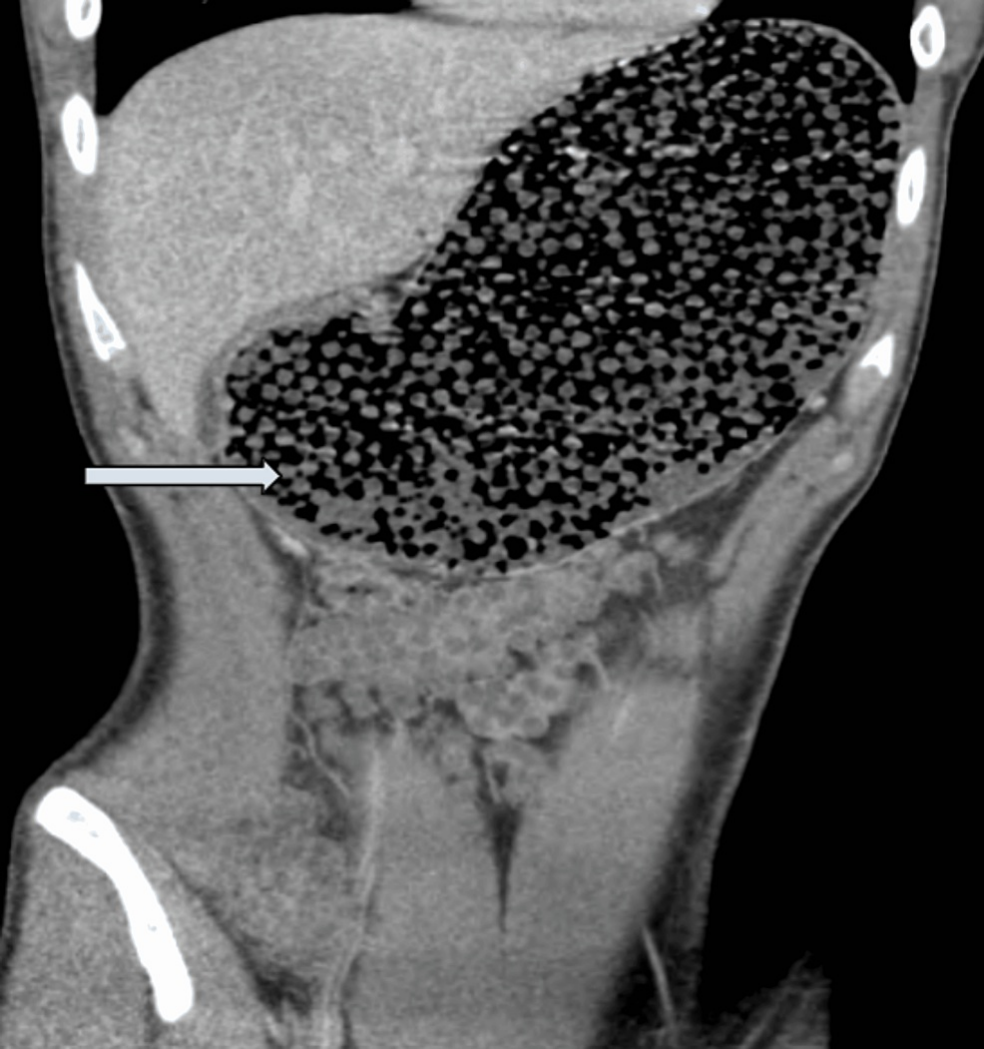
Unenhanced CT scan of the abdomen and pelvis showing innumerable small, rounded densities diffusely throughout the GI tract, most pronounced in the stomach (arrow). GI: gastrointestinal
